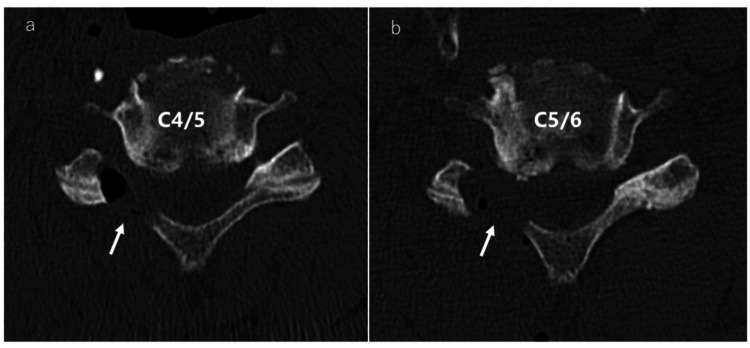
Postoperative CT axial images Posterior decompression is achieved beyond the Luschka joint. The arrows show the decompression sites a: Postoperative CT axial image at C4/5. b: Postoperative CT axial image at C5/6 CT: computed tomography
